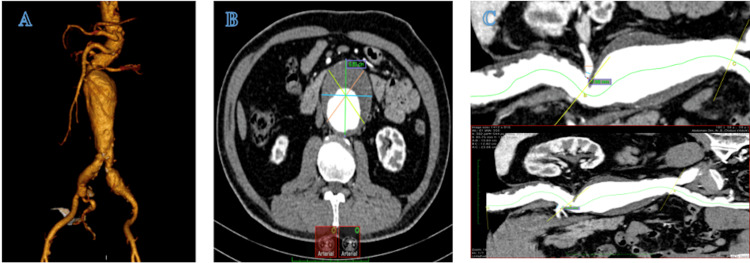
CT scan prior to the procedure showing the anatomical characteristics of the abdominal aortic aneurysm A) Significant short neck and angled aortic neck. B) Largest horizontal diameter of the abdominal aortic aneurysm. C) Stenosis observed in the left renal artery and short length of the right renal artery.
